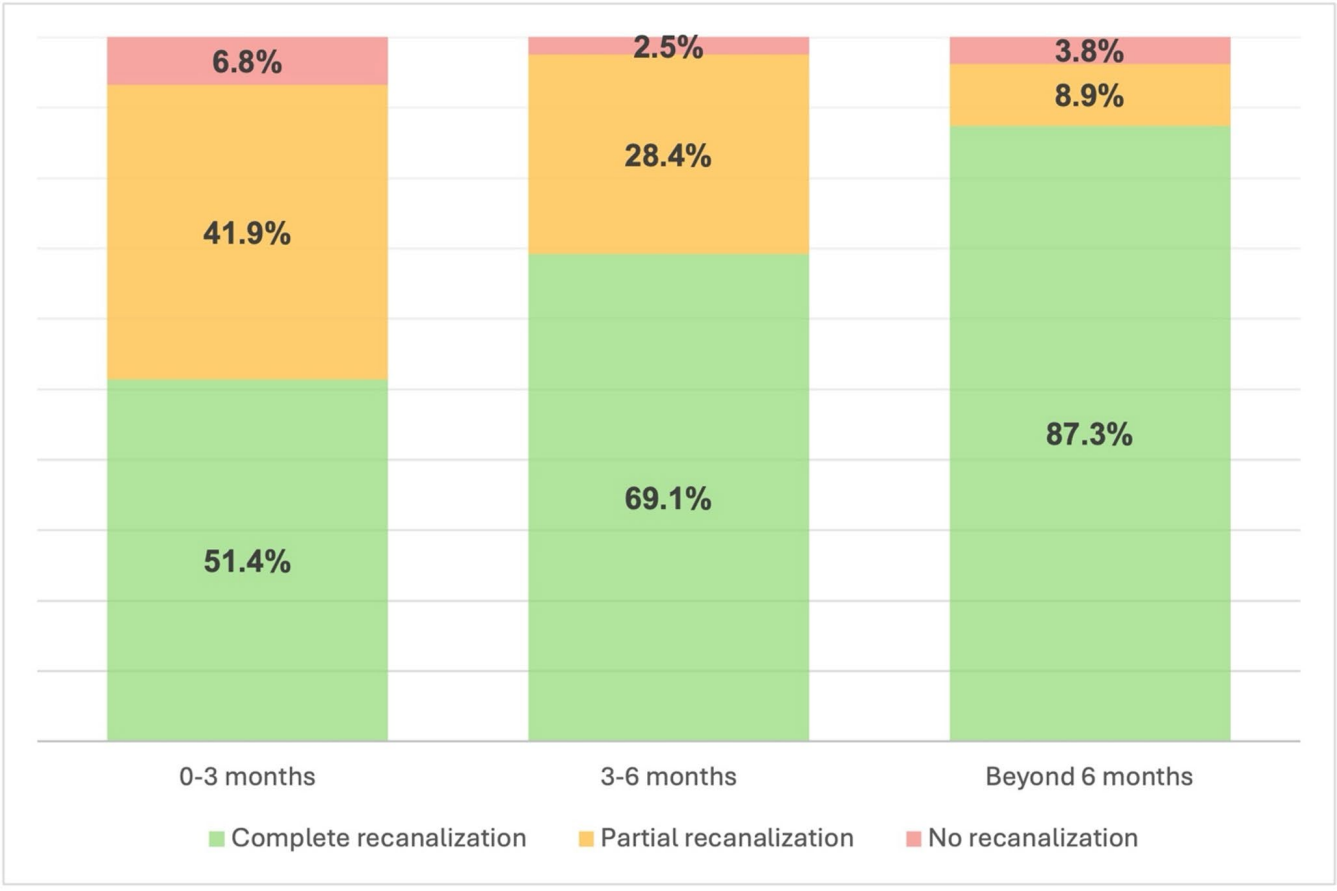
Thrombosis recanalization in the study population assessed by Doppler ultrasound at 3, 6 and beyond 6 months
